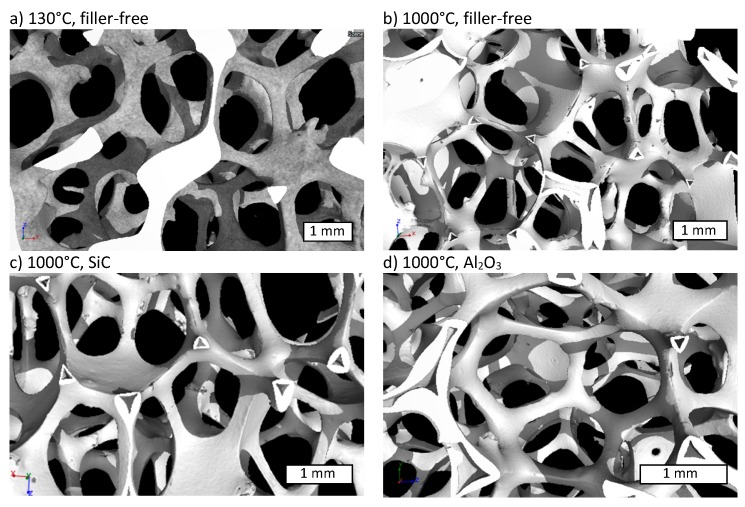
X-ray µ-CT reconstruction image of a volume element representing the typical structure of (a) a crosslinked filler-free foam and (b) of a filler-free foam pyrolyzed at 1000 °C. Foams pyrolyzed at 1000 °C with fillers are shown in (c) with SiC as filler and in (d) with Al_2_O_3_ as filler. The white bars indicate the length scale of 1 mm.
